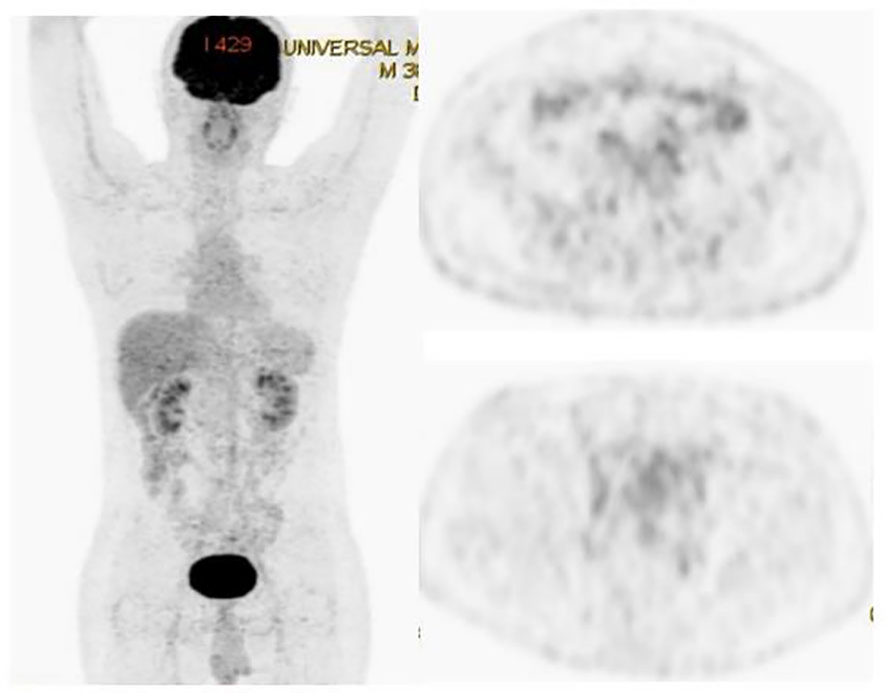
PET-CT showing the remission of all lesions following the treatment.
